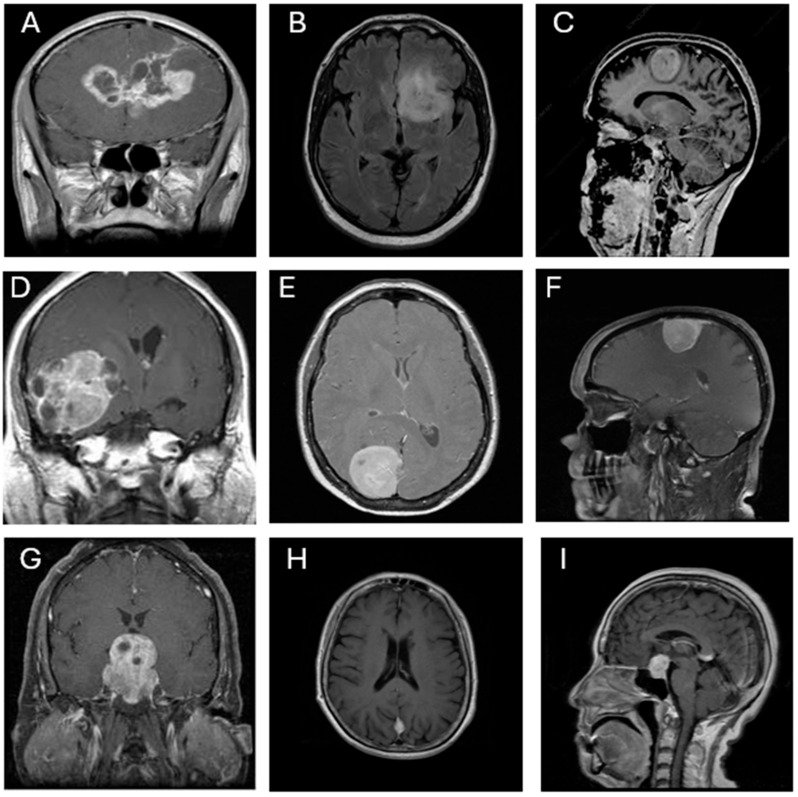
Representative MRI images of three types of brain tumors. (A,B,D) Glioblastoma, (C,E,F,H) meningioma, and (G,I) pituitary adenoma, shown in various orientations (coronal, axial, and sagittal views).
